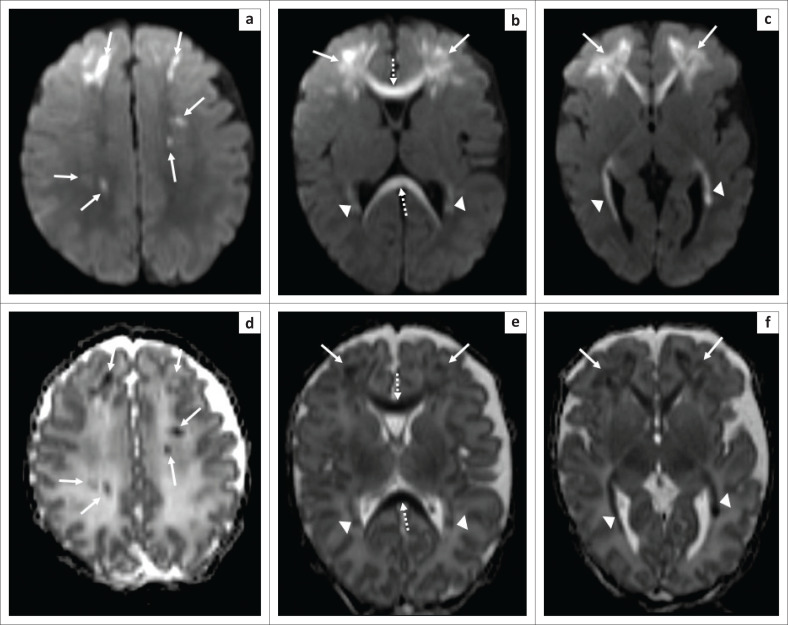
(a, b, c) Axial diffusion-weighted MRI of the brain (repetition time msec/echo time msec, 8500/100; flip angle, 90°; *b*-value = 1000 sec/mm^2^) and (d, e, f) corresponding axial apparent diffusion coefficient maps (8500/100; flip angle, 90°; *b-*value = 1000 sec/mm^2^) at the level of the high frontal lobes (a, d), basal ganglia (b, e) and peritrigonal white matter (c, f). Areas of restricted diffusion involving the bilateral supratentorial white matter, predominantly bilateral frontal lobes (solid white arrows on all images), corpus callosum (dashed white arrows on images [b]) and [e]) and bilateral peritrigonal periventiruclar white matter (solid white arrow heads on images [b], [c], [e] and [f]).
